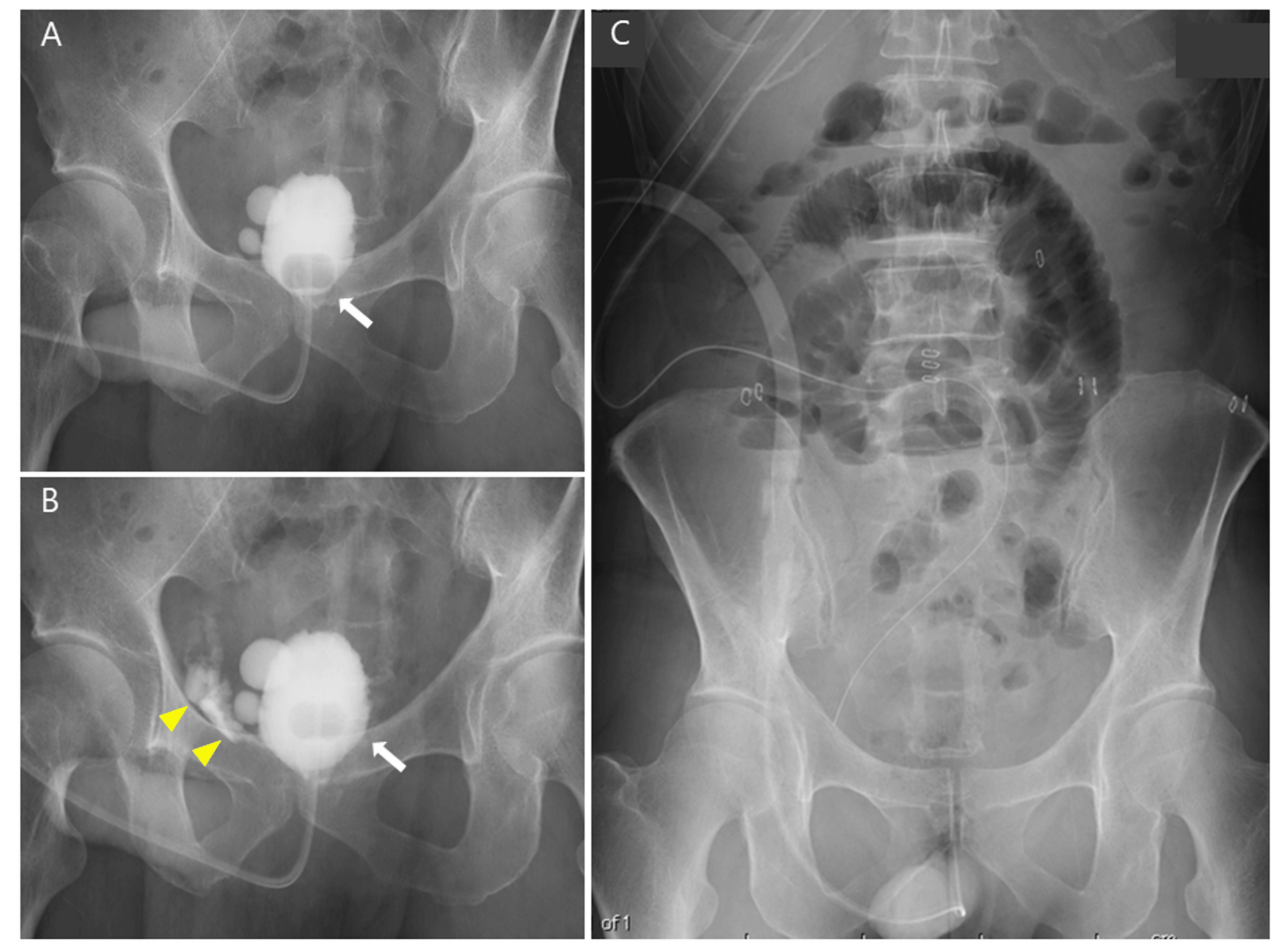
(A) Cystography on POD 2 showing no contrast leakage when gentle traction was applied to the catheter balloon at the anastomotic site. The arrow indicates the location of the catheter balloon. (B) Upon release of the traction, contrast leakage was observed at the 6 o’clock position. The arrowhead indicates the leakage site. (C) Abdominal X-ray on POD 4 demonstrating signs of ileus. POD, postoperative day
